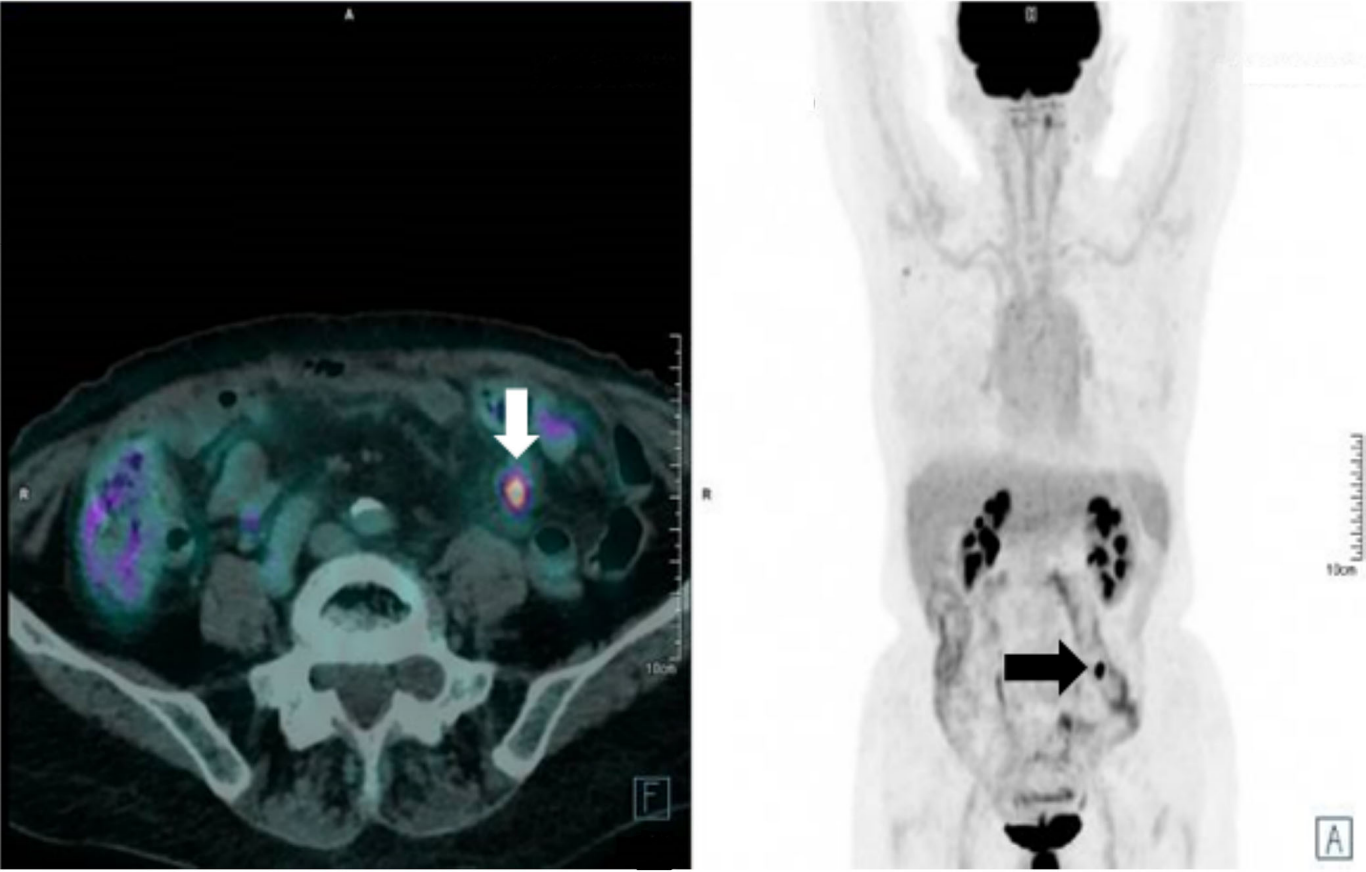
Staging PET-CT used for target definition. The lesion is clearly visible in the hypogastric-left iliac fossa area (see arrows).
